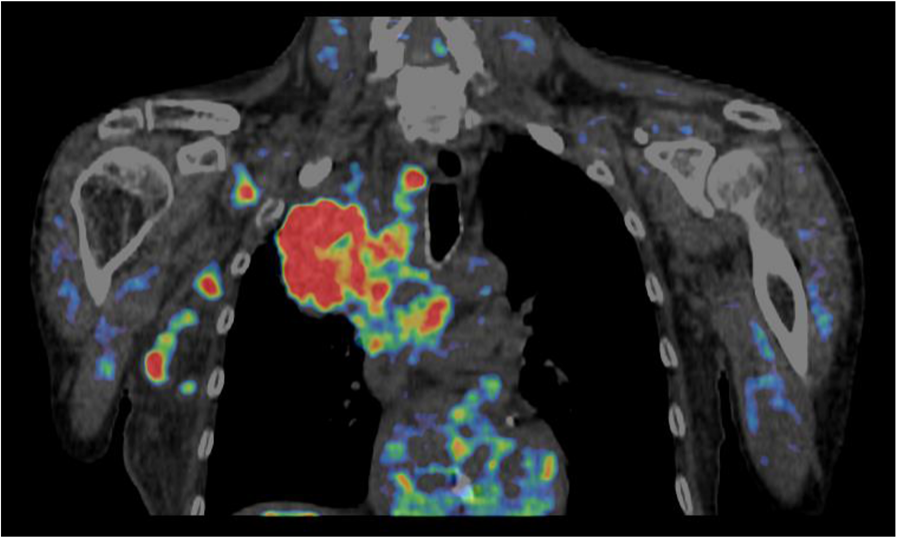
An example coronal FMISO PET image fused with the corresponding CT displayed on a tumor to blood ratio (TBR) color scale. Red regions depict a TBR > 1.4 indicating hypoxia, and no visible PET depicts a TBR < 1, indicating normoxia [4]
